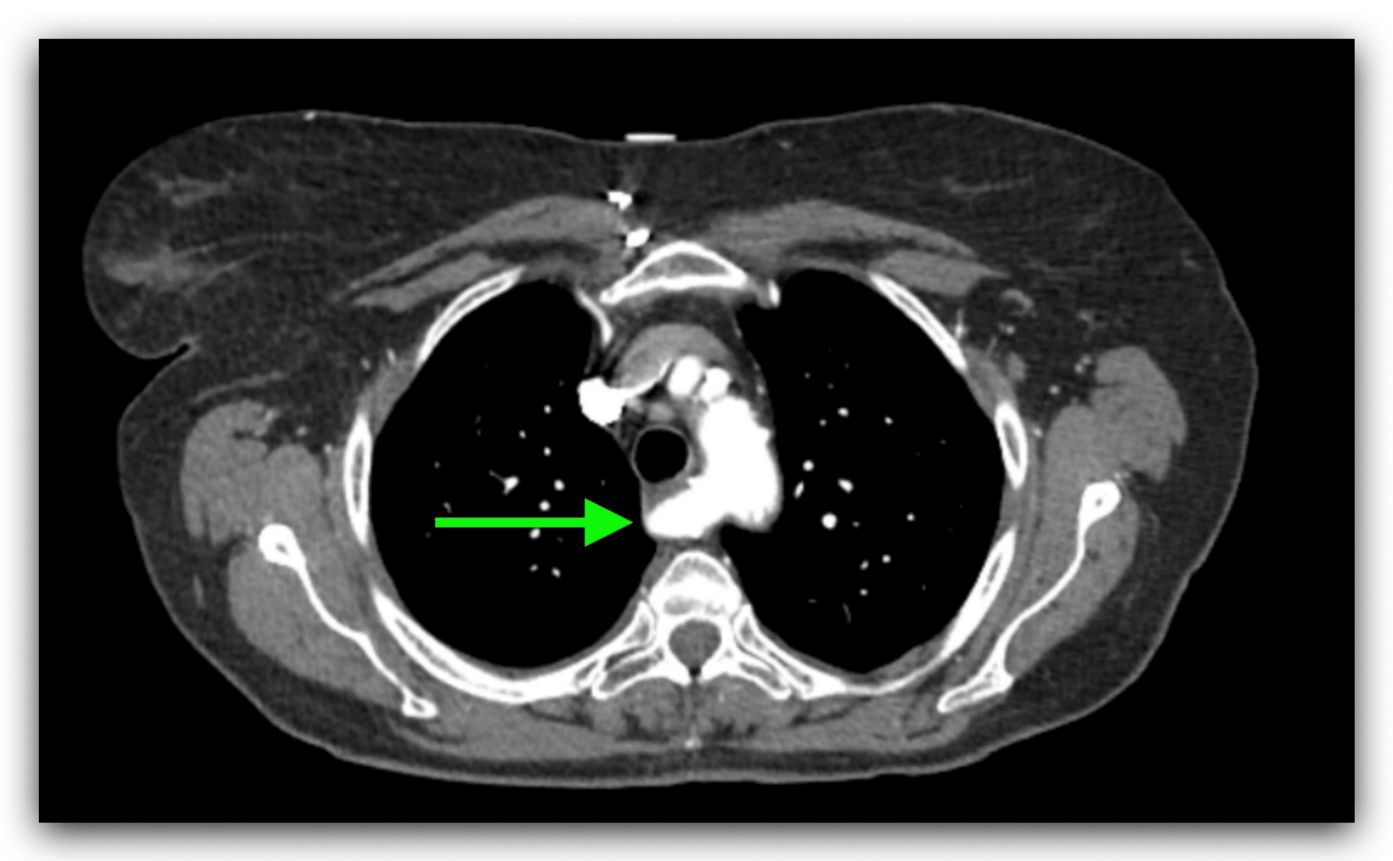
CTA of the chest with contrast showed aberrant origin of the right subclavian artery (green arrow) CTA: CT angiography
